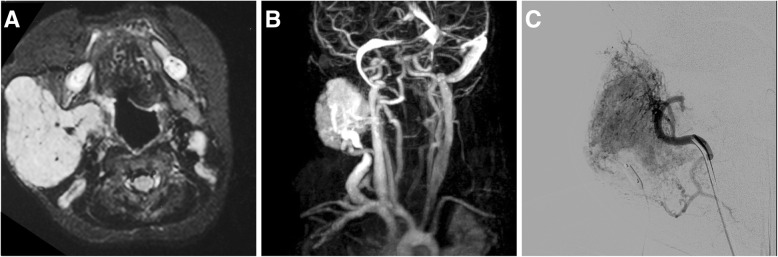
Embolization therapy in large infantile hemangioma with extensive perfusion. In MRI, the hemangioma presents as a rather homogeneous, solid mass with central flow voids (a). Dynamic MR-A shows massive AV shunting during the early venous contrast phase (b). Transarterial particle embolization was performed to reduce the flow and induce a regression with a protection balloon in the draining vein (c)
